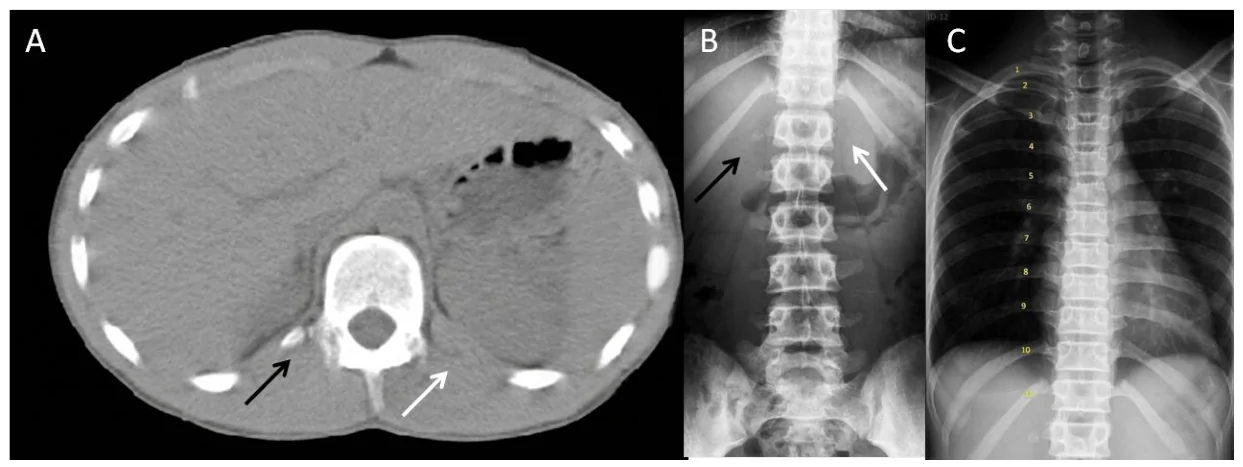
Computed tomography and radiographic evaluation of 12th-rib variations. Panels (A,B) are obtained from a single representative patient; the axial CT image (A) and the correlated anteroposterior radiograph (B) demonstrate a hypoplastic right 12th rib (indicated by black arrows) and a concomitantly absent left 12th rib (indicated by white arrows). Panel (C) is obtained from a separate patient, showing a frontal radiograph highlighting bilateral costal variations for comparison.
